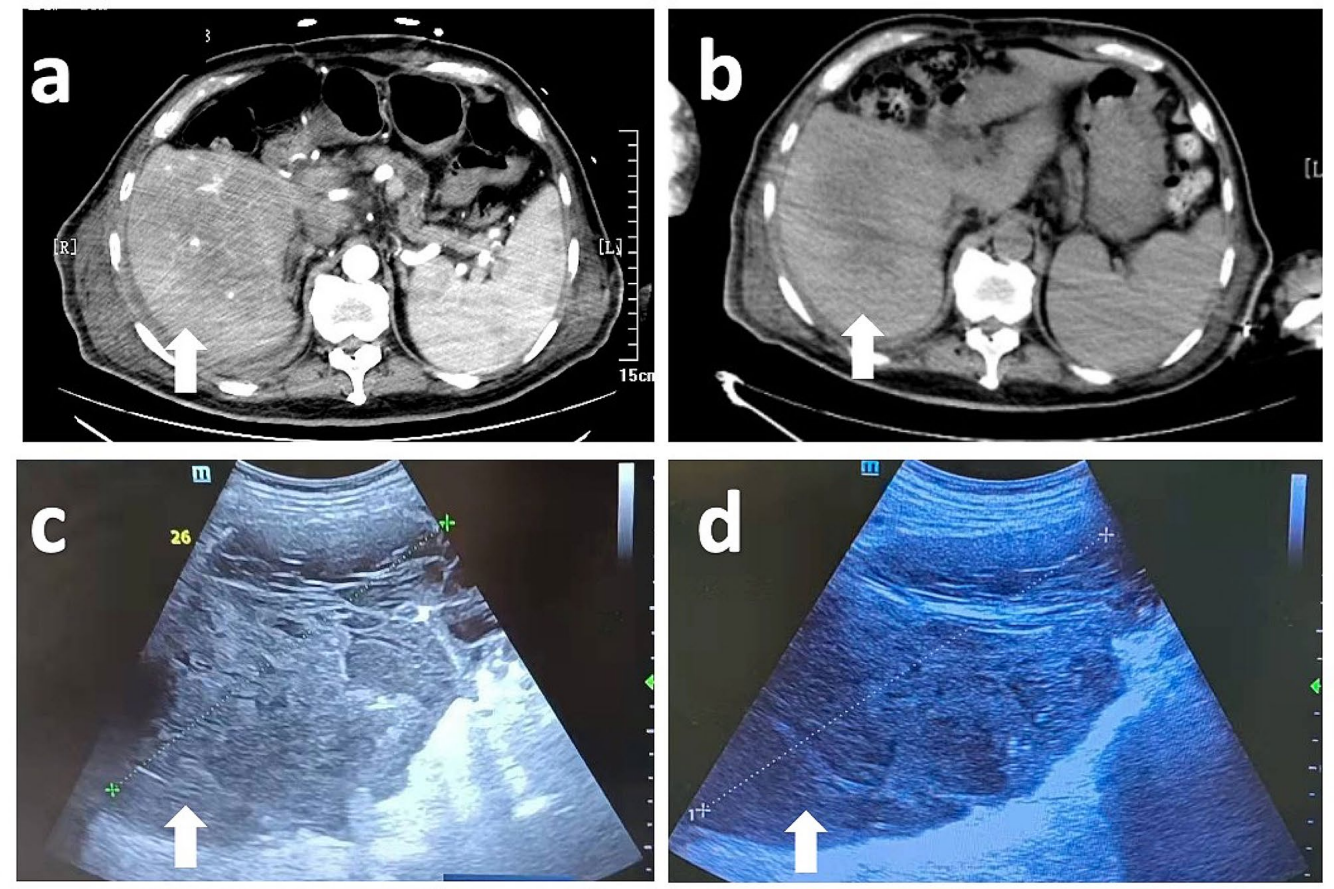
Computed Tomography images of the patients’ hematomas. This image shows the 14.5*11*11.3 cm hepatic hematoma and the 24.7*12.6*12.6 cm retroperitoneal hematoma (white arrows)
